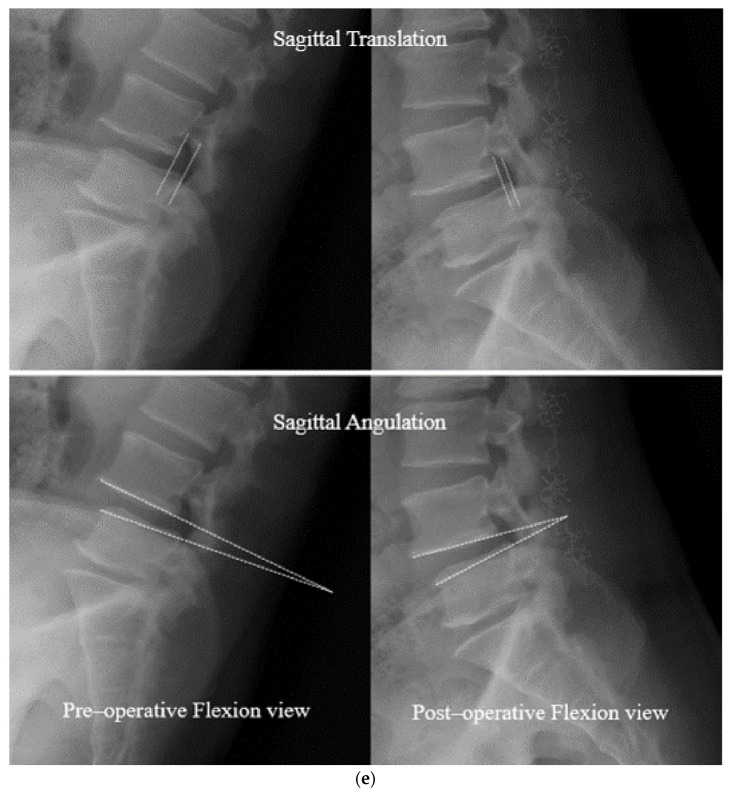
Case 1. An 82-year-old male patient suffered from low back pain, sacral pain on both sides, and numbness in both legs for 3 years. (a) Preoperative MRI and myelogram: spinal stenosis at L1 to L5. (b) Preoperative dynamic X-ray: instability at L4–5. (c) Postoperative MRI: well-decompressed dural sac. (d) Postoperative dynamic X-ray: instability improvement at L4–5. (e) Sagittal translation decreased from 9.1 mm to 5.2 mm at L4–5. Sagittal angulation increased from 5.8° to 11.4°. Source: own composition. The small white dot line represents an extension of the posterior wall of the vertebra body and indicates the degree of listhesis. The large white dot line is an extension of the endplate line of the vertebra body and represents the degree of angulation.
